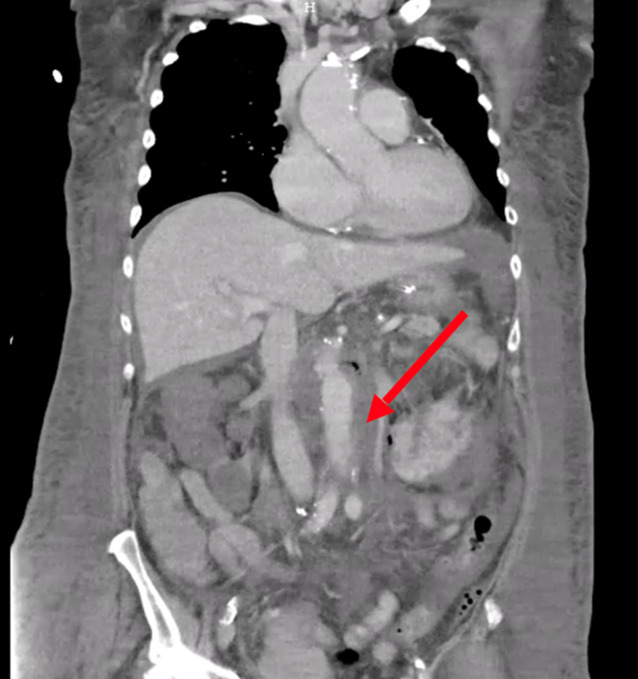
Postoperativer Kontrollbefund (CT, koronare Rekonstruktion) des infrarenalen MBAA nach OR und Implantation einer Xenograft-Prothese – Fall 1. Der *rote Pfeil* markiert die ursprüngliche Position des resezierten Aneurysmas (Quelle: Klinik für Radiologie und Nuklearmedizin, Universitätsklinikum Magdeburg A. ö. R.)
